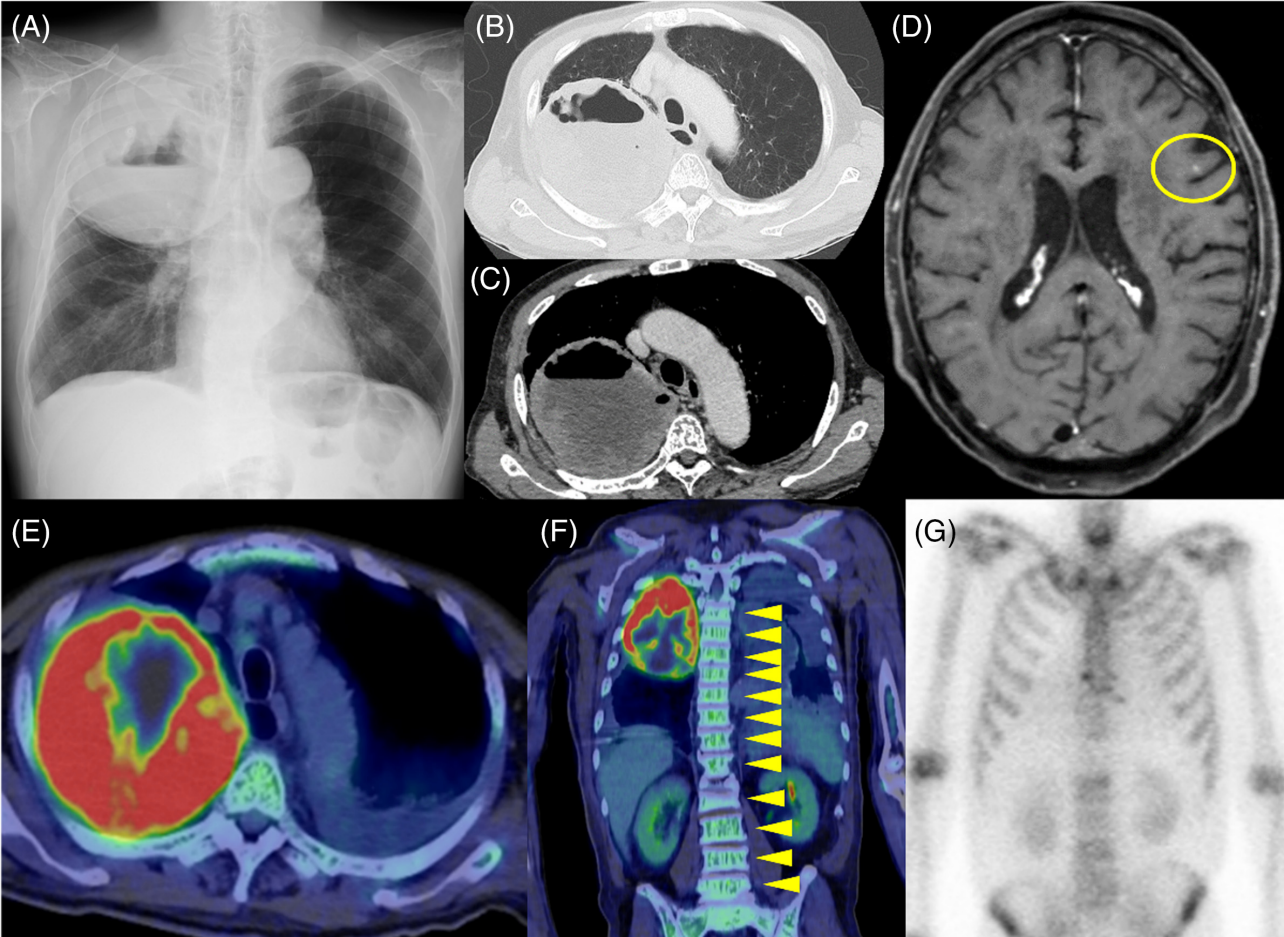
Image inspection on admission. (A) Chest radiograph revealed an 11 cm tumour shadow in the upper right lung field. It was formed with a cavity. (B) and (C) Chest contrast‐enhanced computed tomography revealed a mass in the right upper lobe. It was formed with a cavity and internal liquid storage. (D) Magnetic resonance imaging T2‐weighted images showed a tumour in the left frontal lobe (yellow circle). (E) 18F‐fluorodeoxyglucose positron emission tomography/computed tomography (18F‐FDG‐PET/CT) revealed elevated uptake in the mass in the right upper lobe, with a maximum standardized uptake value max of 18.0. (F) 18F‐FDG‐PET/CT revealed diffuse uptake in the bone marrow (yellow arrowheads). (G) Bone scintigraphy revealed no significant uptake in the spine.
